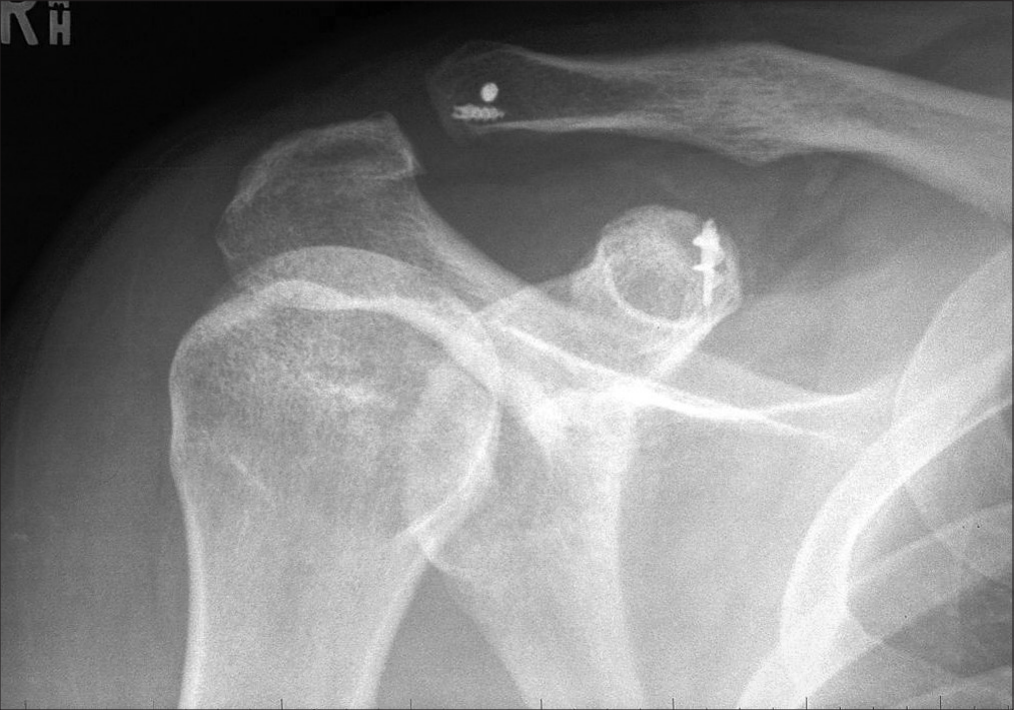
Radiograph of the shoulder 6 months after surgical stabilization using suture anchors, showing satisfactory reduction of the acromioclavicular joint
